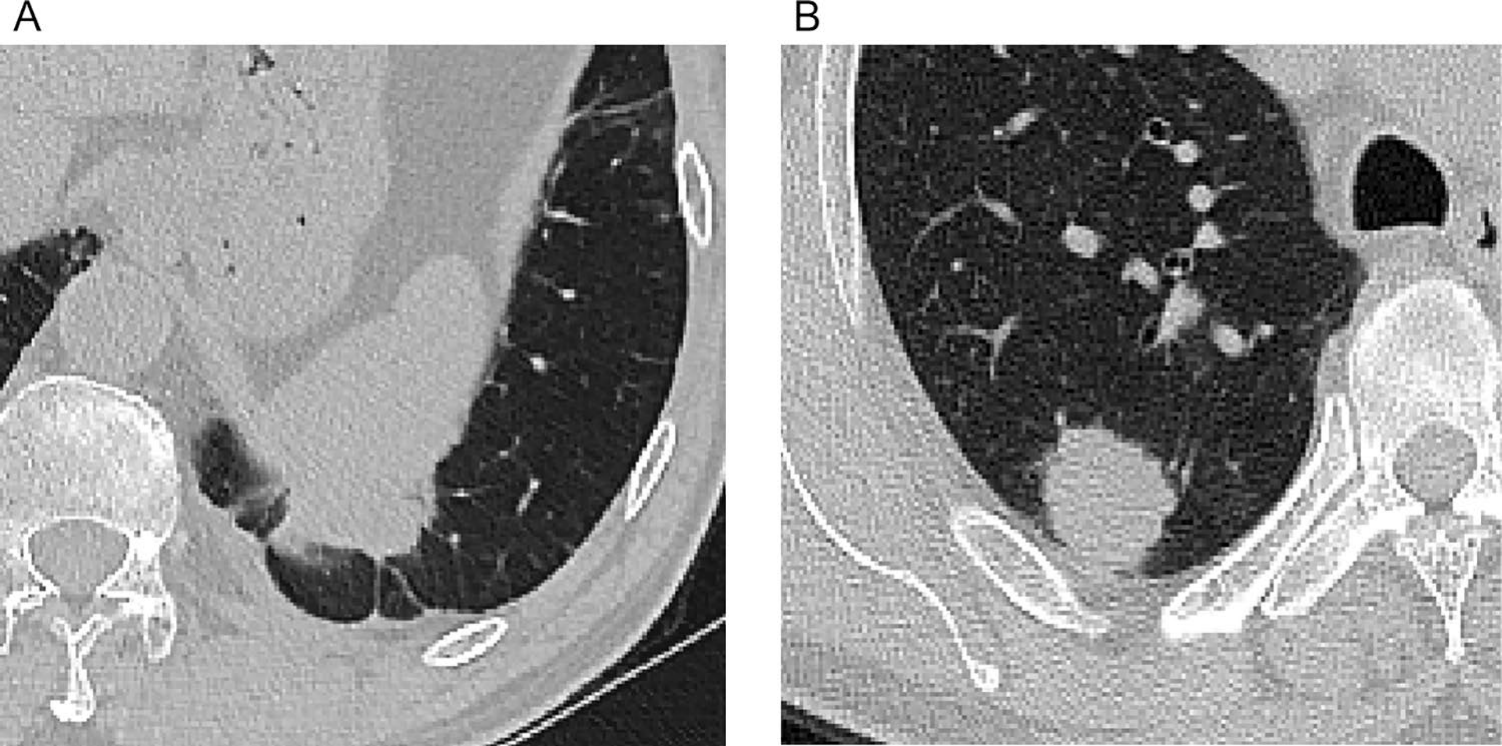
A In a case of lung adenocarcinoma with VPI, the CT scan lung window image reveals the tumor's positioning beneath the pleura, with pleural attachment and a long pleural contact length characterized by an obtuse angle of contact. B Conversely, in a case of lung adenocarcinoma without VPI, the CT scan lung window image depicts the tumor located beneath the pleura, with a shorter curve length and an acute angle of contact
